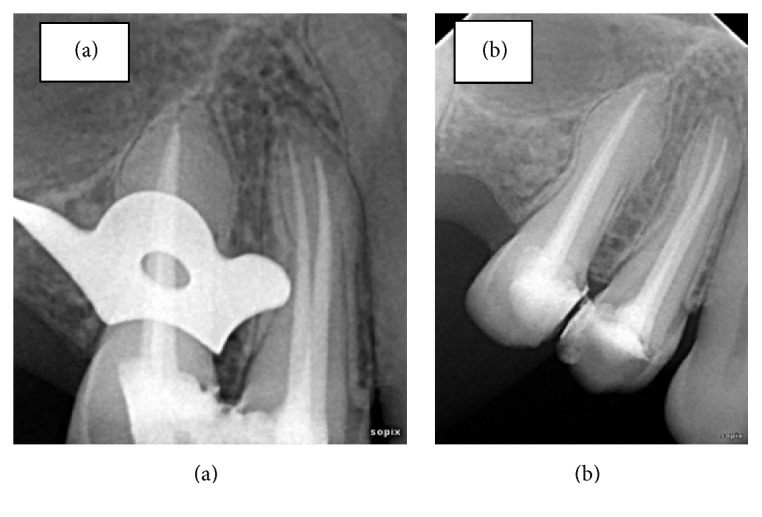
(a) and (b) showing obturation and 6-month follow-up radiographs, respectively.
